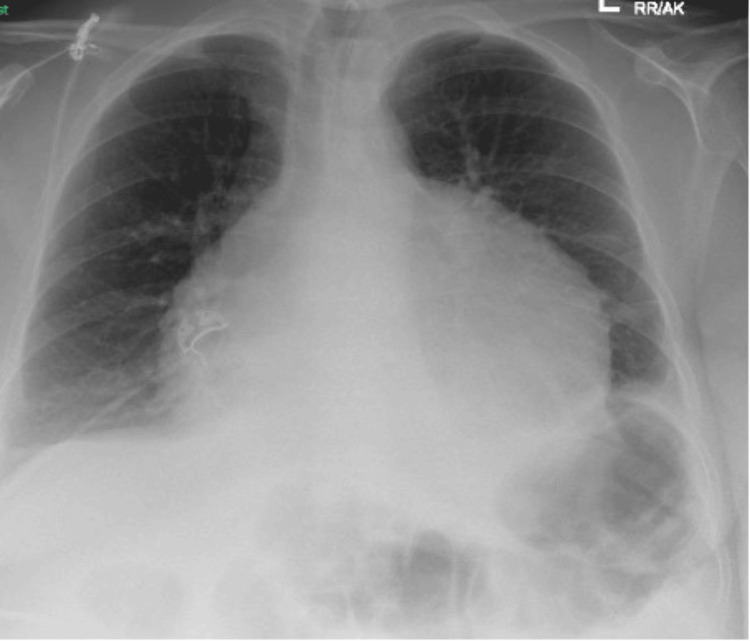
The chest X-ray shows an enlarged heart. There are small effusions at both costophrenic angles and upper lobe blood diversion, and minimal opacity at the right base and in the lingula, which suggest a degree of left heart failure.
